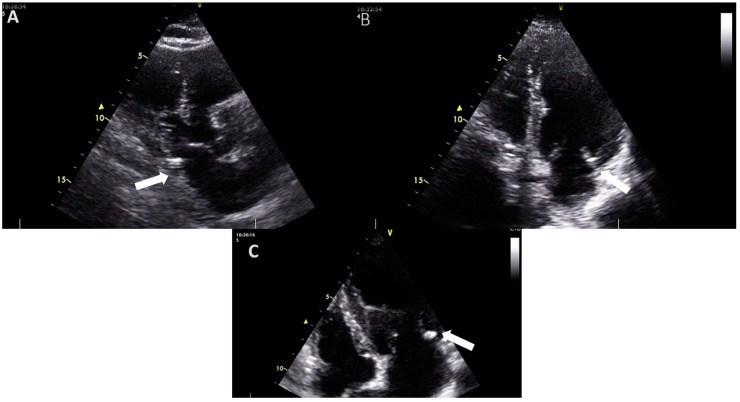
The appearance of mitral annular calcification on transthoracic echocardiography ((A), transthoracic parasternal long axis view (B), and (C) transthoracic apical 4-chamber view). It appears as an echogenic structure at the base of the posterior leaflet (white arrow). Images are from the personal collection of Professor Floria Mariana.
